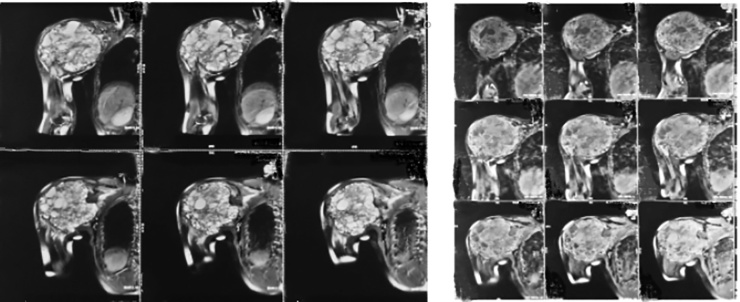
Magnetic Resonance Imaging showing an expansile lytic lesion.
